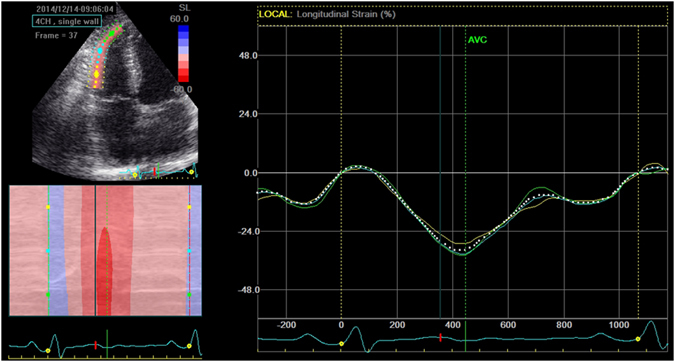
RV longitudinal strain curve by 2D STE. RV, right ventricle; 2D STE, two-dimensional speckle-tracking echocardiography.
